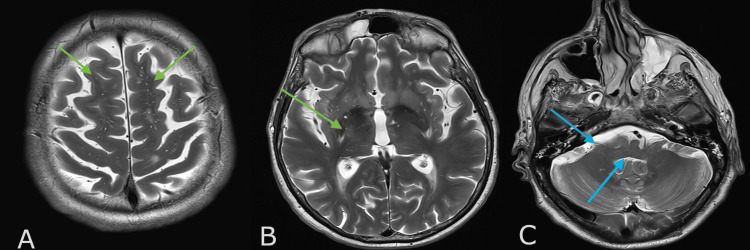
Magnetic resonance imaging of the brain following stabilisation of blood pressure (A), (B), and (C): T2 Weighted images showing near complete resolution of the cortical/subcortical signal in the cerebral hemisphere (green arrows in Figure A), thalami (green arrows in Figure B), brainstem, and cerebellum. In comparison to the previous images, CSF spaces and cisterns were normal with no mass effect (blue arrows in Figure C).
